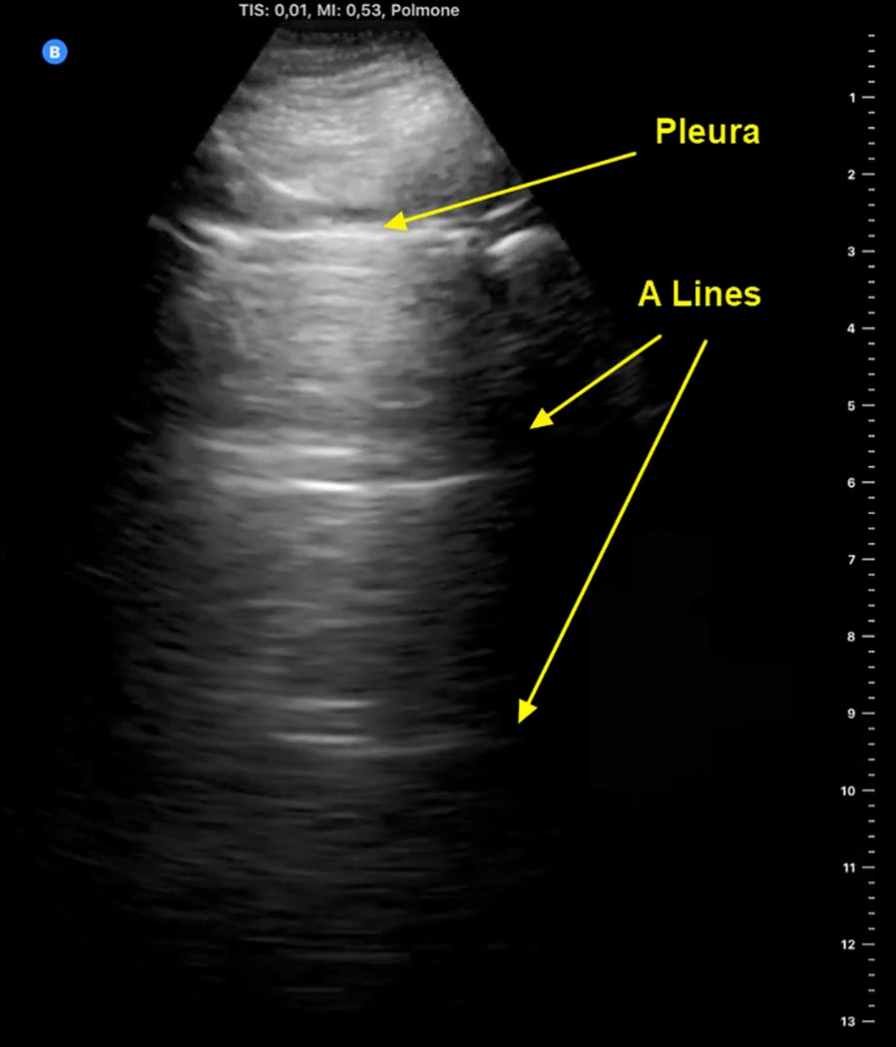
Anterior parasagittal lung ultrasound scan of the thorax. The parietal and visceral pleura appear as a hyperechoic line with sliding movements (also see Additional file 1: Clip S1). A lines are thought to be mirror artifacts from the near-total ultrasound reflection by the pleura-alveolar gas interface
